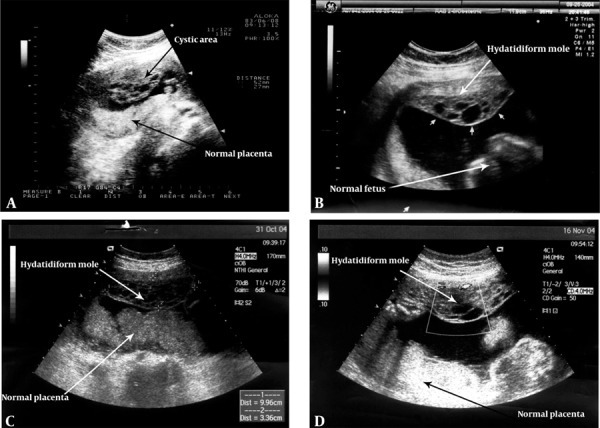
Ultrasonography in a 39-year-old woman with twin pregnancy with a complete hydatidiform mole and a coexisting viable fetus; A, US shows a cystic area at the anterior side of the uterus and normal placenta at 15 weeks of pregnancy; B, Ultrasonography shows a normal fetus with hydatidiform mole at 18 weeks gestation; C, Ultrasonography shows a normal placenta and fetus with hydatidiform mole at 24 weeks gestation; D, Ultrasonography shows placenta and fetus with hydatidiform mole at 28 weeks gestation.
